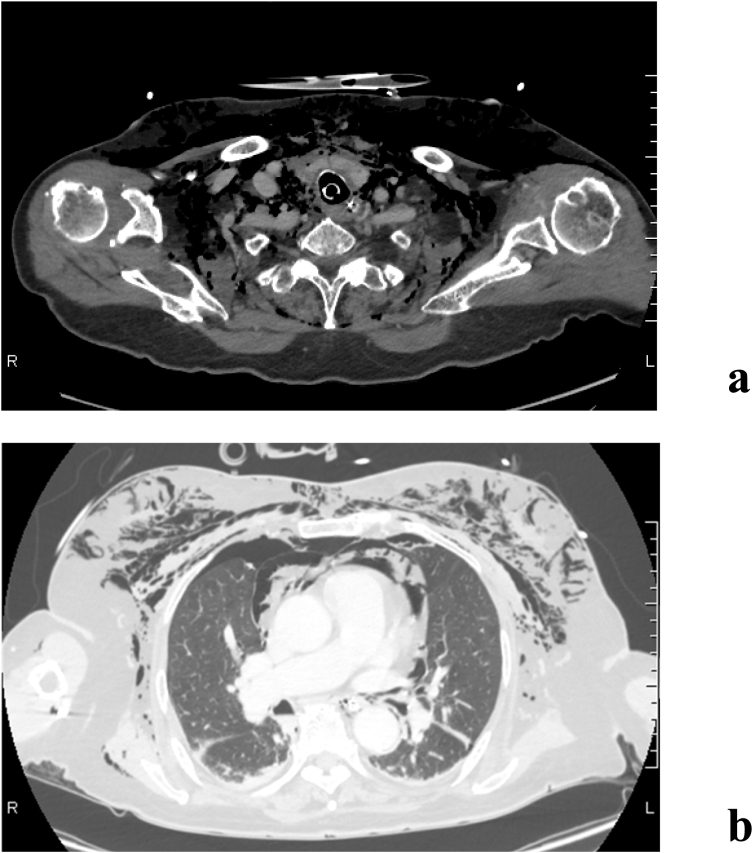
Enhanced computed tomography performed on admission showing the thyroid injury (a) and subcutaneous emphysema, mediastinal emphysema, and bilateral pneumothorax (b).
